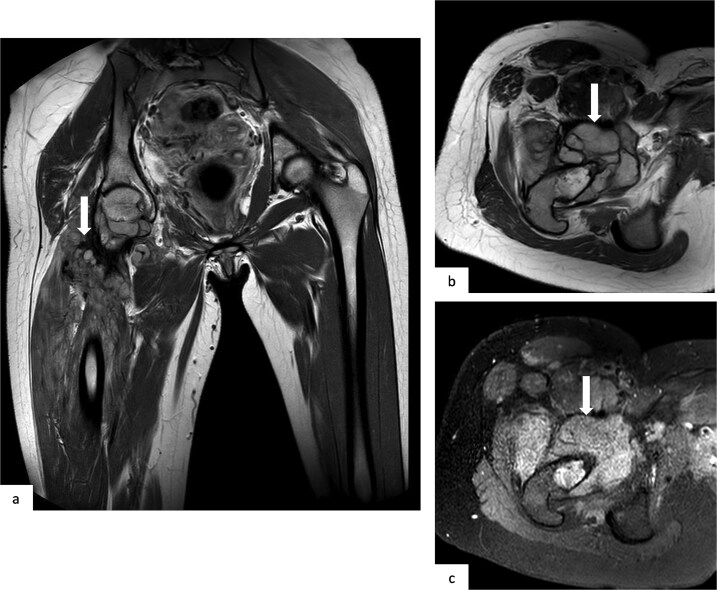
Coronal T2-weighted MR image (a), axial T2-weighted MR image (b), and axial T1-weighted fat-saturated MR image after gadolinium injection (c). MRI of the right thigh demonstrates a tumor centered on the femoral neck (arrows), showing high signal intensity on T2-weighted images (a,b) and heterogeneous enhancement after contrast administration (c). The lesion invades the coxofemoral joint and extends into the anterior muscle compartment.
